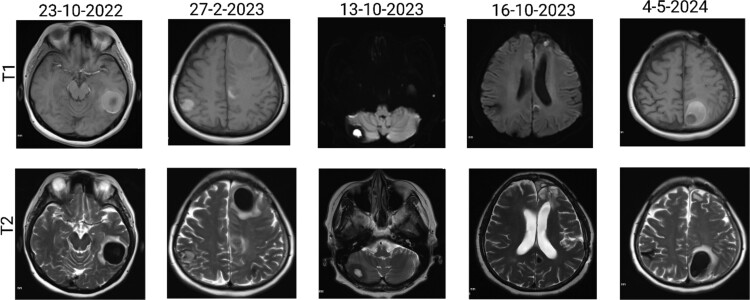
Brain MRI showing dynamic haemorrhage. (a) T2-weighted MR image showing multiple CCMs. The highlighted portions indicate areas of hemosiderin deposition associated with previous haemorrhages. (b) post-contrast T1-weighted MR images showing the enhancement patterns of CCMs and the extent of the surrounding oedema.
